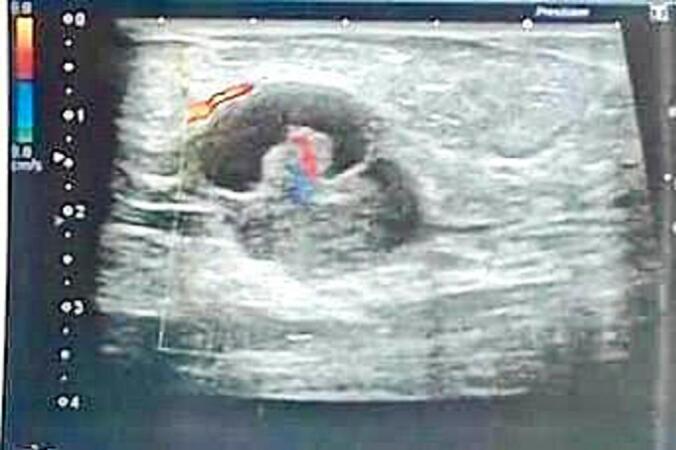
Ultrasonography imaging Cystic dilatation of an intraductal canal centred by a tissue materiel measuring 26.5mmx13.6 mm, categorized according to BIRADS system (Breast Imaging Reporting and Data System (BI-RADS) category 4c).
